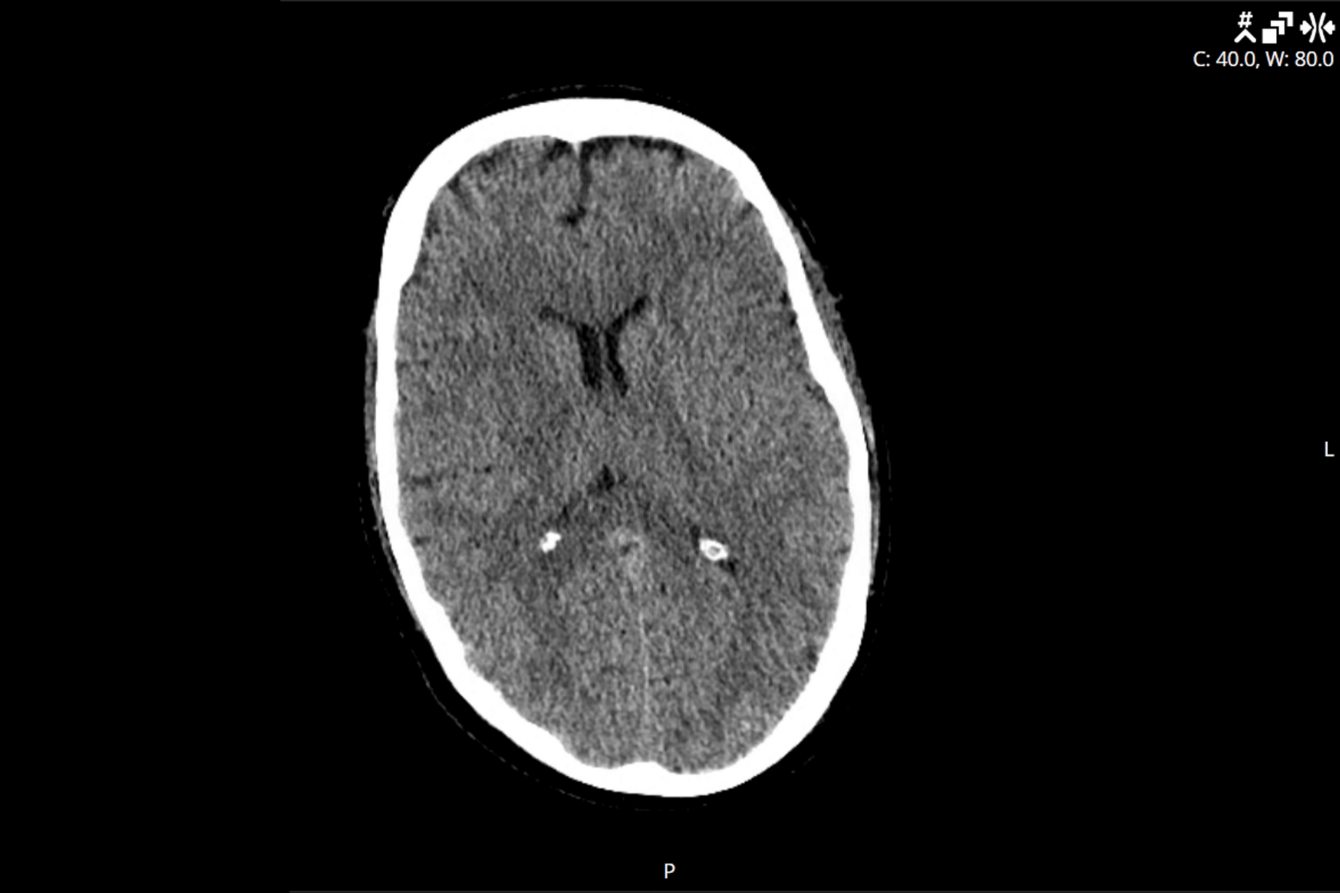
Noncontrast axial CT imaging of the brain with normal findings CT: Computed tomography.
